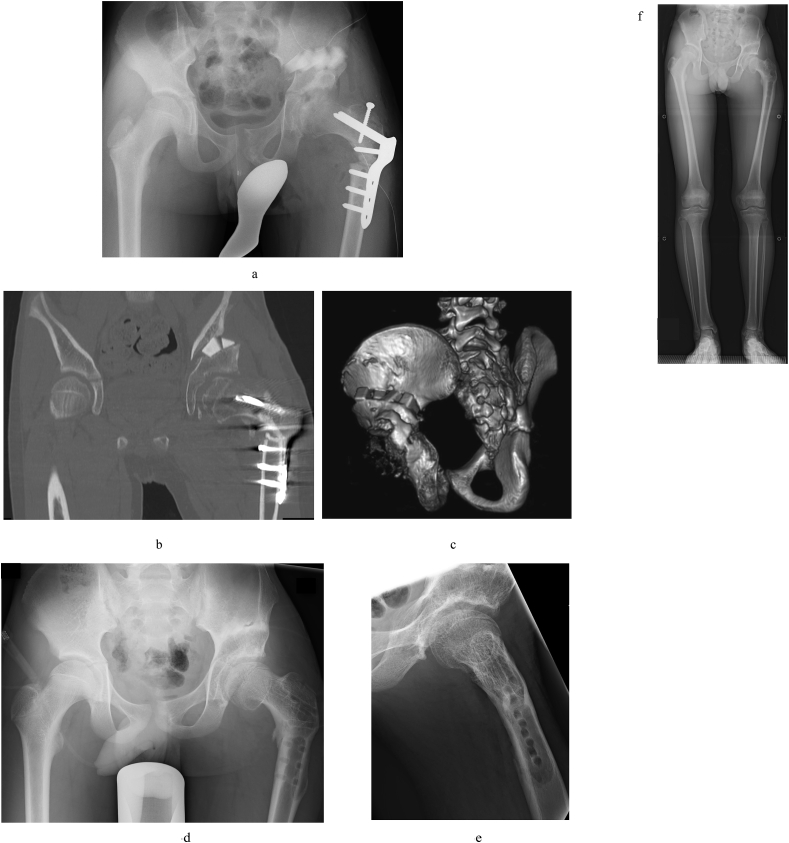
a) X-ray taken immediately after surgery. b) Postoperative CT coronal section. The femoral head has reduced, and acetabular roof coverage is good. c) Postoperative three-dimensional CT showing that artificial bone has been inserted into the osteotomy site. d) and e) X-ray taken at 1 year after surgery. f) Full-length X-ray of the bilateral legs at 2 years after surgery. A difference between the length of the legs persists.
